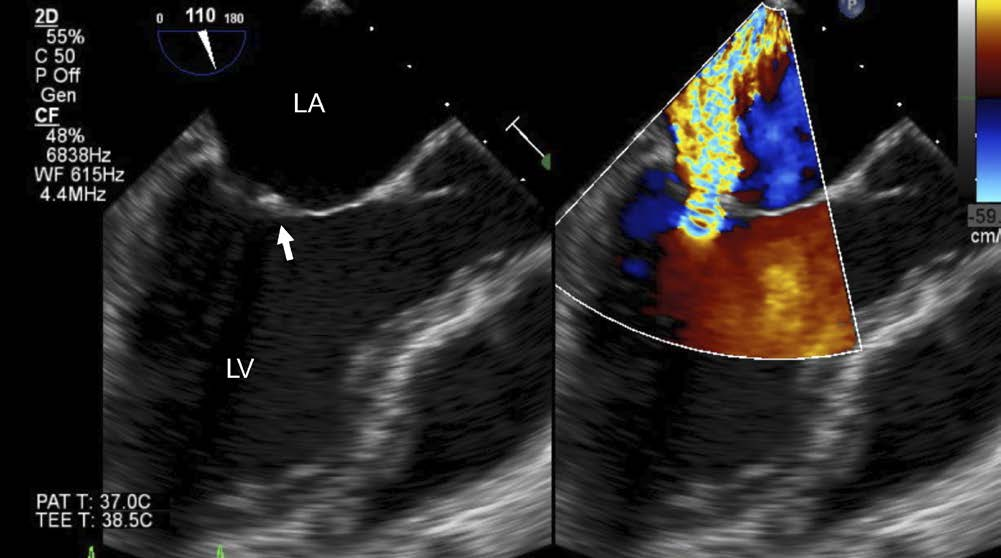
Severe mitral regurgitation in a patient with systemic lupus erythematosus with non-bacterial endocarditis. The patient was a 42-year-old woman with SLE and double-factor positive antiphospholipid antibody syndrome. Mitral regurgitation and endocarditis were suspected, and she was admitted to the hospital as an emergency. TEE showed severe mitral regurgitation and non-bacterial verrucous vegetations at the anterior leaflet (white arrow). LA = left atrium, LV = left ventricle, SLE = systemic lupus erythematosus, TEE = transesophageal echocardiography.
